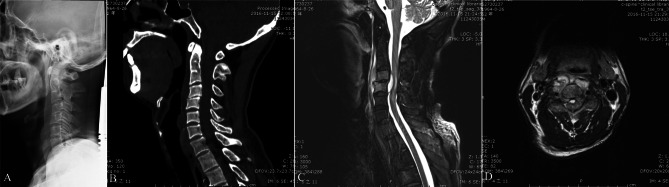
(A) Lateral radiograph shows loss of C4-5 intervertebral disc height. (B) Sagittal computed tomography image shows no sign of bone destruction. (C, D) Magnetic resonance imaging scans show the formation of paravertebral and epidural abscesses and spinal cord compression at the C4-5 level
